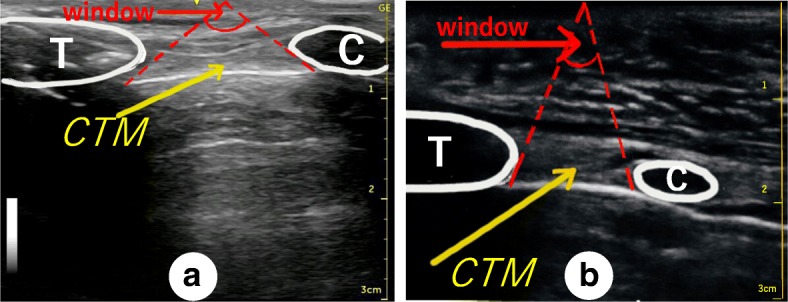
Cervical ultrasonographic images obtained using the longitudinal approach. Either the transverse or longitudinal approach can be used for ultrasonographic identification of the cricothyroid membrane (CTM). Using the transverse approach, the operator manipulates the ultrasound probe while tilting it up and down on the patient’s neck to locate the CTM; if the operator is attempting to locate a deeply positioned CTM (a), the angle of tilt of the probe may be restricted, and is shown as a window (a, red lettering). In contrast, if the CTM is in a shallow position (b), the angle of tilt of the probe may be wider using the transverse approach. However, there is no need to tilt the probe when using the longitudinal approach. a An ultrasonographic image of the patient’s neck using the longitudinal approach. b An ultrasonographic image of the first author’s neck using the longitudinal approach. The first author is a healthy male adult with a standard physique (height 174 cm, body weight 68 kg). T, thyroid cartilage; C, cricoid cartilage; CTM, cricothyroid membrane
